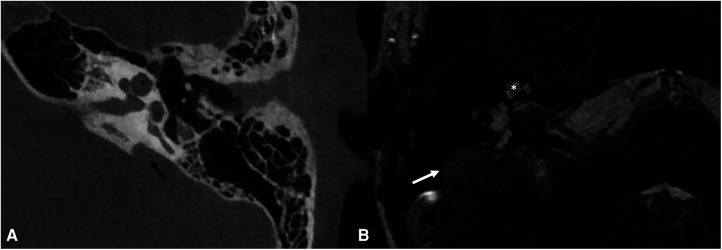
Bone-CT (A) imaging of a case of left incomplete partition type II and enlarged vestibular aqueduct (black arrow). T2 weighted-MRI (B) imaging of a case of right incomplete partition type II with enlarged endolymphatic sac (white arrow). Note in both images the presence of the upper part of the cochlea that appears to be globose (asterisks). The incomplete partition type II and the enlarged vestibular aqueduct are frequently both present in the same patient.
